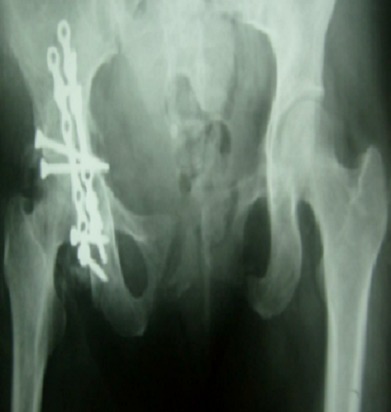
Radiographie de contrôle après traitement par double voie d'abord antérieure et postérieure
